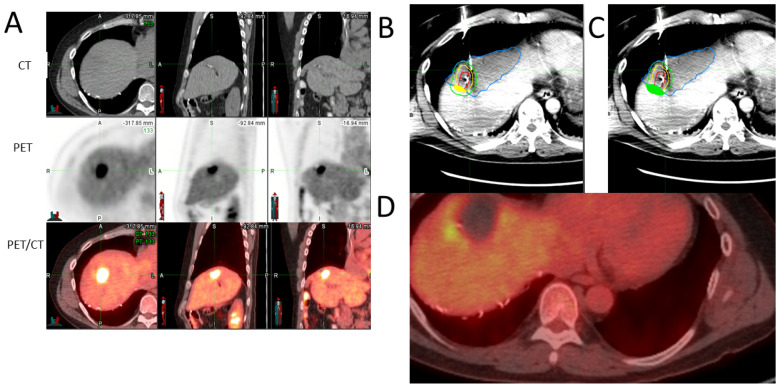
Importance of accurate tumor and margins detection for preventing local tumor progression. A patient with colorectal liver metastasis in segment8. (A) Pre-ablation anatomical imaging (CT) and PET and fused PET/CT. The CLM was detected on PET and PET/CT but not easily detectable on CT alone. Insufficient coverage of margins (5 mm margin filled with yellow (B) and 10 mm margin filled with green (C)) led to tumor progression (D) in less than 6 months after thermal ablation in the area where the tumor was not sufficiently covered.
